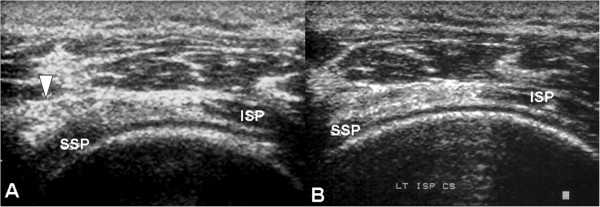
Supraspinatus tendon attrition before and after implants removal. Sonographic findings of a 40-year-old man who had shoulder impingement syndrome before implant removal. (A) A partial thickness tear was noted at the bursal aspect of the supraspinatus tendon (SSP), which resulted from repetitive mechanical attrition by the hook of the plate (arrow). The infraspinatus tendon (ISP) was intact. (B) 1 month after implant removal, the same patient was re-evaluated. Musculoskeletal sonography showed the dimpling lesion had become less obvious than before.
